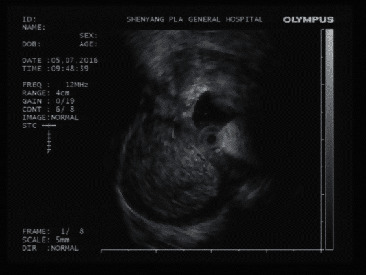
Endoscopic ultrasonography showing a mass-like lesion with outward growing muscularis propria. Heterogeneous and dense punctate hyperechoic foci were found in the lesions, the thick borders were clear, and it showed echo signals like muscle.
